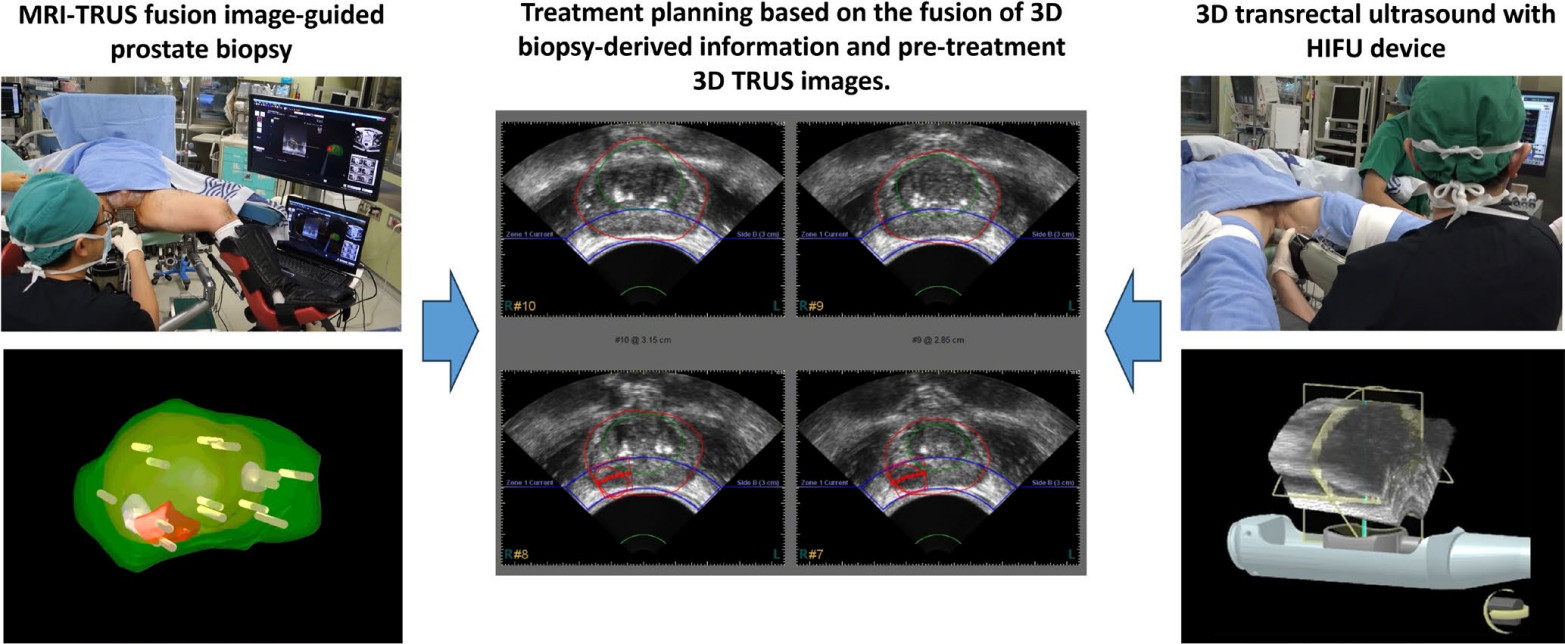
Magnetic resonance imaging‐transrectal ultrasound (TRUS) fusion image‐guided treatment planning in focal therapy using high‐intensity focused ultrasound. In commercial high‐intensity focused ultrasound (HIFU) devices for prostate cancer (Sonablate), treatment planning utilizes high‐resolution TRUS images. To achieve precise localization of the treatment area, three‐dimensional (3D) cancer localization data from preoperative MRI‐TRUS fusion image‐guided biopsies were integrated with 3D ultrasound images from the HIFU device. This fusion enables the visualization of the target area in a two‐dimensional format, assisting in accurate and effective treatment planning.
